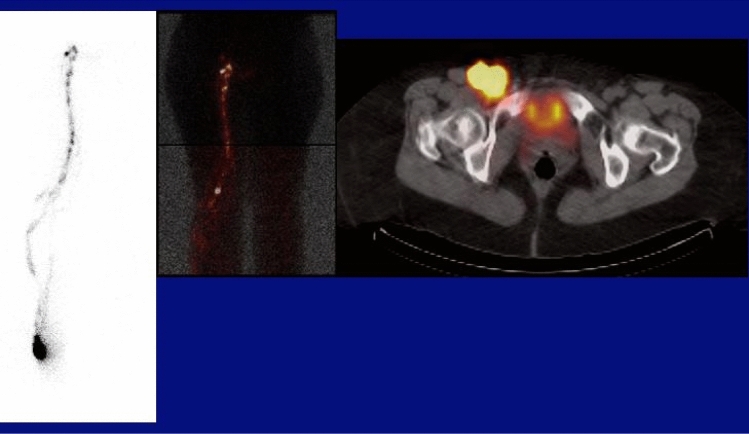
Lymphoscintigraphy with the injection site at the lateral right ankle. Activity is visualized in multiple lymphatic vessels in the right lower extremity on the partial whole-body acquisition (left). Delayed images fused with transmission images to show the body outline (middle) demonstrate a popliteal sentinel node in addition to multiple right inguinal nodes. SPECT provides cross-sectional images that can be registered to CT showing the “hot” right femoral SLN (right)
